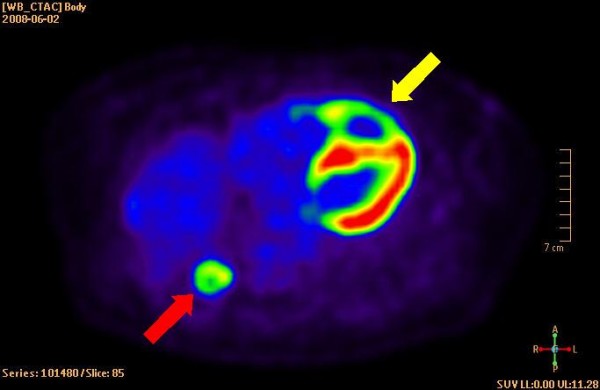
Display of PET-image. The yellow arrow indicates the metastasis of the leiomyosarcoma. Note the absence of FDG uptake in the center of the tumor, thus suggesting necrosis. The red arrow indicates of the lung metastases.
